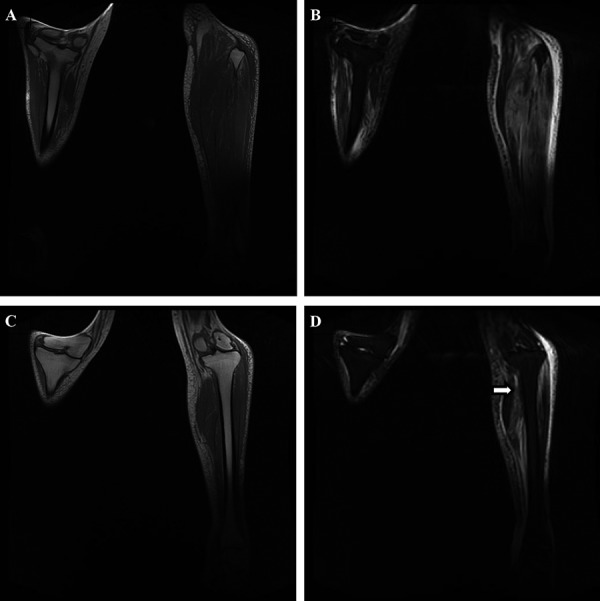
Coronal T1-weighted (A & C) and T1-weighted fat-suppression technique known as short tau inversion recovery (STIR) (B & D) MR images of the knee and upper leg reveal perifascial, and intramuscular edema within the left calf muscle, interestingly sparing the medial head of the gastrocnemius (D = white arrow). There is increased signal intensity from the lateral head of the gastrocnemius and lateral compartmental muscles of the lower leg (B & D). The asymmetric distribution of the findings and the involvement of noncontiguous muscles are characteristic of this condition.
